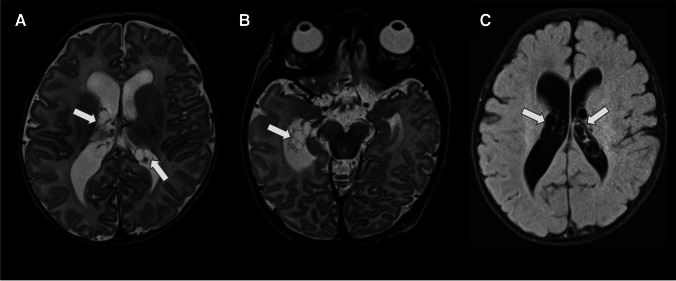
Choroid plexus cysts (white arrows) in a newborn. A, B Axial T2-weighted images display multiple small cysts in both lateral ventricles. C The axial FLAIR image demonstrates that the cysts have a signal similar to that of the CSF
